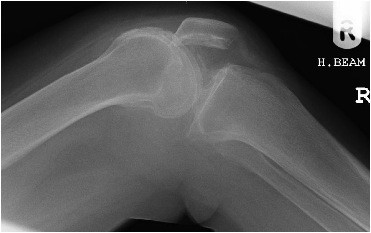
Plain lateral view X-ray obtained at the time of admission.
